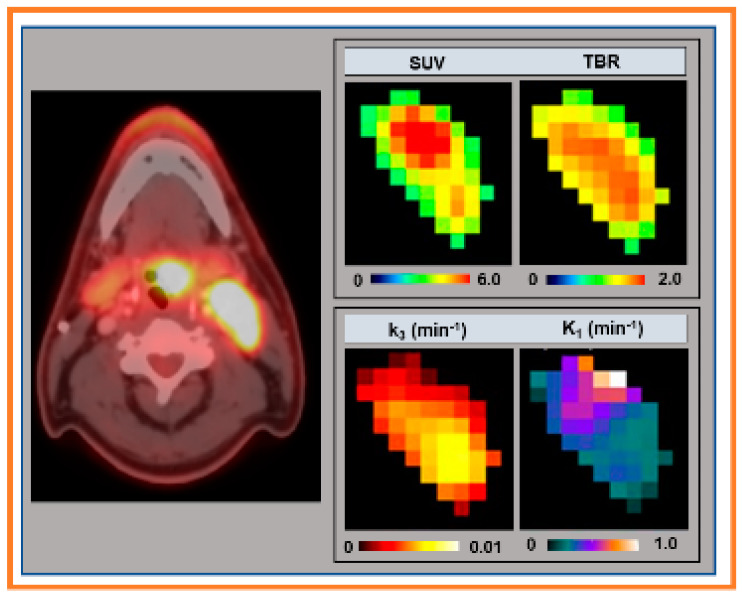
Left column: Representative axial images of ^18^F-fluoromisonidazole (^18^F-FMISO) positron emission tomography (PET/CT) from HPV-positive head and neck squamous carcinoma patient. Right column: ^18^F-fluorodeoxyglucose-PET/CT derived standard uptake value (SUV) and FMISO-PET derived tumor to blood ratio (TBR), k_3_, and K_1_ maps.
